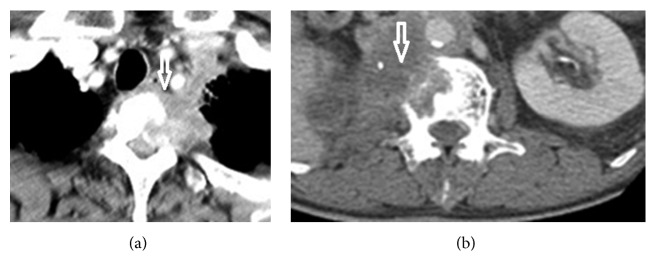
Primary tumor spread directly to the vertebral body. CT scans showing (a) lung squamous cell carcinoma and (b) large renal pelvis urothelial carcinoma that spread directly to the anterolateral portion of the vertebra. Arrows indicate lesions and spreading routes within the vertebral body.
